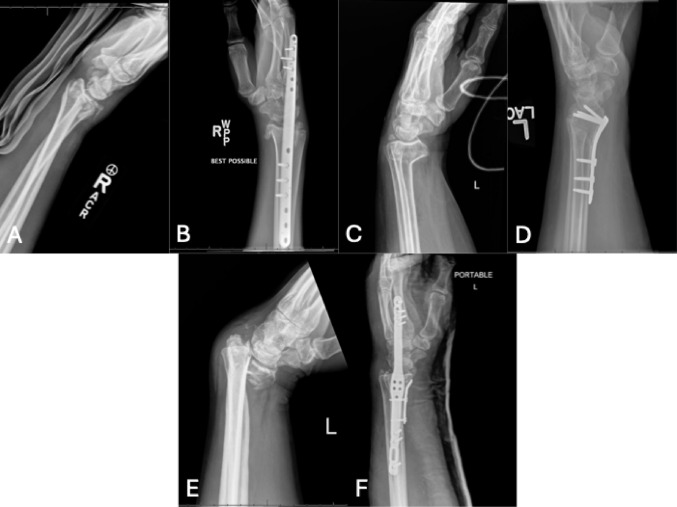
Representative radiographic examples of fixation constructs used for AO/OTA 2R3C distal radius fractures. A Injury radiograph of a right distal radius fracture. B Postoperative radiograph demonstrating dorsal bridge plate fixation. C Injury radiograph of a left distal radius fracture. D Postoperative radiograph demonstrating volar plate fixation. E Injury radiograph of a left distal radius fracture. F Postoperative radiograph demonstrating combined dorsal bridge plate and volar plate fixation
